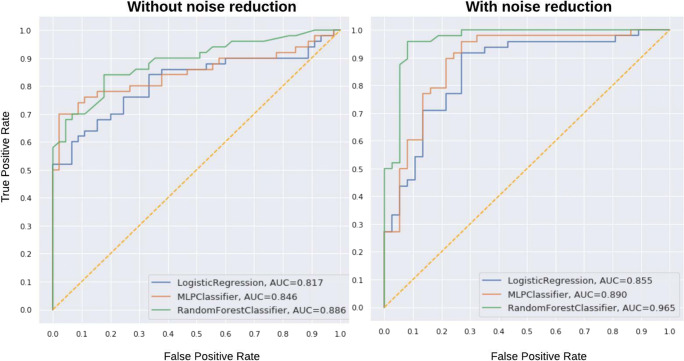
The receiver operating curves of machine learning algorithms showing their ability to classify normal chest CT from other CO-RADS stages on the hold-out dataset (scenario 1). The performance of the classifiers increased after noise reduction
